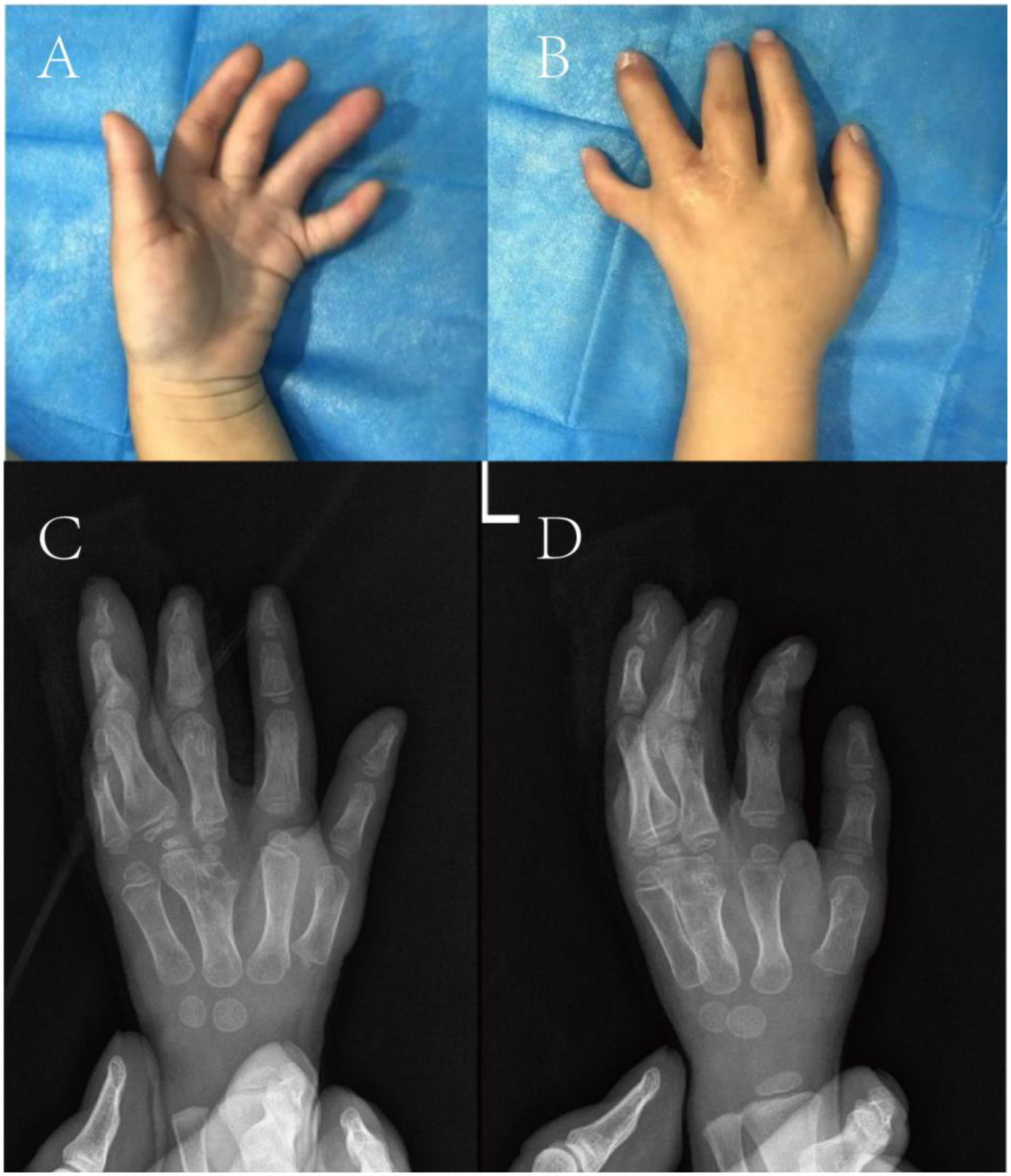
Follow-up pictures at one year after the operation. (A,B) Appearance photographs. (C,D) x-ray films.
